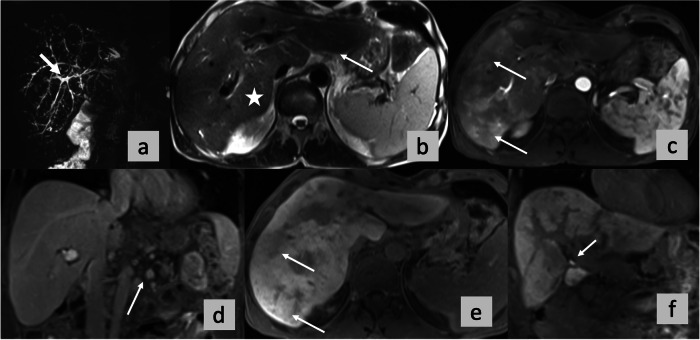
No functional stricture (NFS). OLT for HD. A 55 years-old male PSC patient. Coronal maximum intensity projection from 3D MRCP (a), axial T2-weighted image (b) and post-contrast T1-weighted images with fat suppression in arterial phase (c, axial) and portal venous (d, coronal) and HB phase (e, axial and f, coronal). ANALI_Gd_AP, ANALI_Gd_HBP, and ANALI_NoGd_.scores were calculated by all 5 readers. Readers A-E graded gadoxetic acid-enhanced AP images as 2, and HBP images as 2. In other words, all readers registered parenchymal enhancement heterogeneity as present in the AP (⇥ in c), as well as in the HBP (⇥ in e). But on non-contrast images, ANALI_NoGd_ scores were 4,4,3,3,2 for readers A, B, C, D, and E, respectively. Reader A, B and D scored the IHBD as 1 (4 mm, ⇥ in a), Reader C and E as 0 (≤ 3 mm, ⇥ in d in a). Reader A, C, and D rated the liver as deformed = 1 (⇥ in d in b), whereas readers B and E judged the liver as normal = 0. Reader E scored no signs of portal hypertension = 0, whereas, due to collateral vessels (⇥ in d), all other readers scored PH as = 1. All 5 readers called no functional stricture (NFS) since contrast excretion is seen within the intra- and extrahepatic bile ducts in the HBP (⇥ in d, f)
